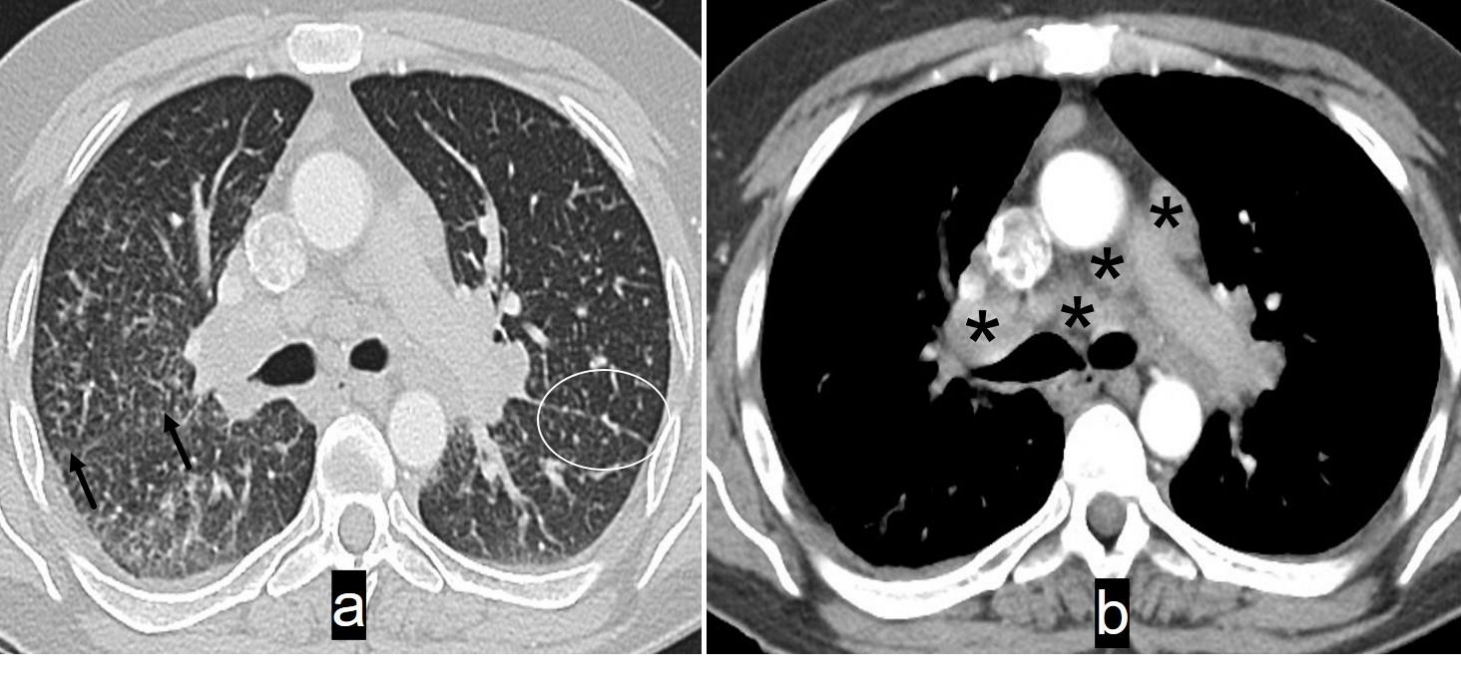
Contrast enhanced CT of a 31 years old female with sarcoidosis. Lung window (a) shows widespread reticulonodular opacities in both the lungs. Note presence of small nodules along the left oblique fissure (circled) suggesting peri-lymphatic distribution of nodules - a hallmark of sarcoidosis. Right oblique fissure is largely obscured by the opacities, but its probable location is indicated (arrows). Mediastinal window (b) shows multiple enlarged lymph nodes (asterisks) in the pre-carinal, pre-vascular and right hilar regions
